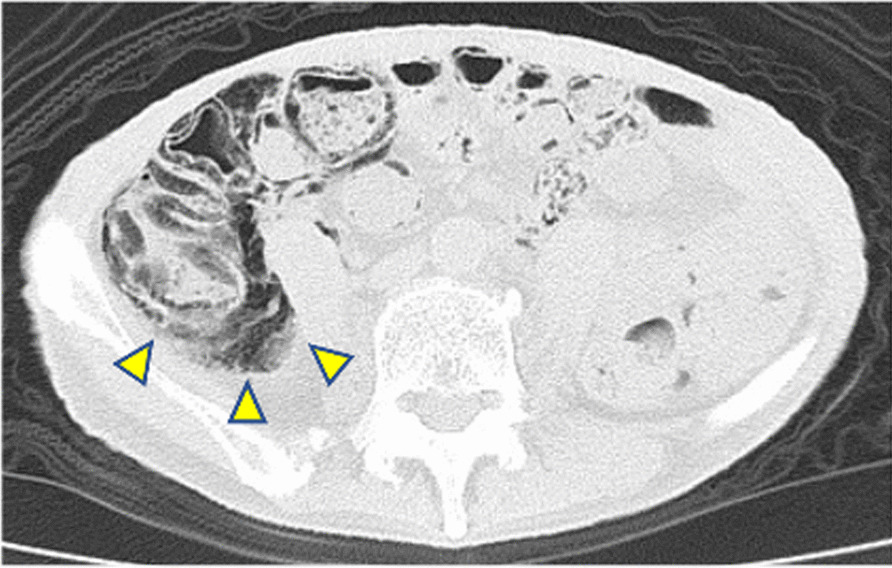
CT image taken 14 weeks after the introduction of lenvatinib showing pneumatosis intestinalis of the ascending colon. The arrows are pointing to emphysema in the intestinal wall of the ascending colon
